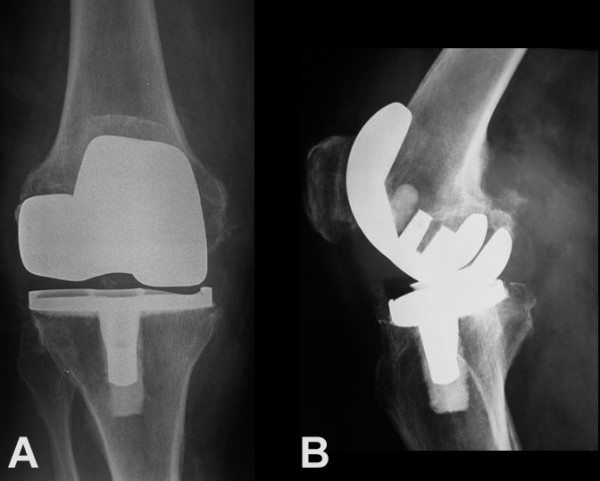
(A) An anteroposterior radiograph of the right knee shows narrowing of the medial joint compartment, varus malalignment of the prosthetic joint, and radiolucent lines around femoral and tibial components. (B) Lateral radiograph shows a soft tissue mass at the popliteal fossa.
